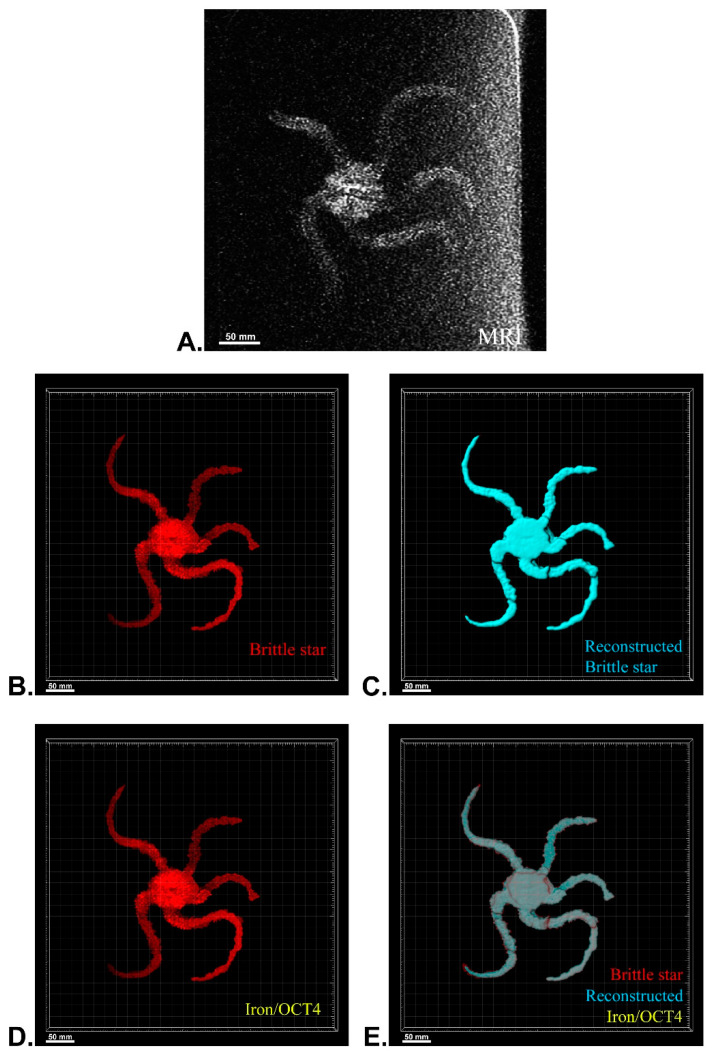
Three-dimensional (3D) reconstruction of brittle star tissue with no treatment using magnetic resonance imaging (MRI) serial images: (A) original grayscale image; (B) whole tissue imaging and signals of the brittle star tissue without reconstruction; (C) brittle star whole tissue 3D reconstruction in which the blue surface represents reconstructed brittle star tissue; (D) 3D reconstruction of the iron/iron plus anti-Oct4 signals, which is negative in this image; (E) 3D reconstruction of the whole tissue of brittle star along with all signals. All scale bars in four images are 50 mm. All images were processed and generated by Imaris software (V 7.4.2, ImarisX64; Bitplane AG, Schlieren, Switzerland).
